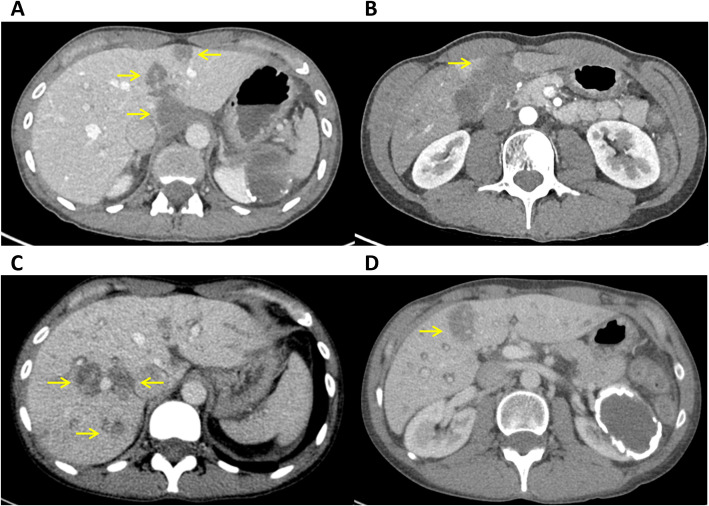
(A, B) Axial contrast-enhanced CT scans acquired within 24 hours after the first and (C, D) after the second histotripsy treatment session showing expected response in the treated lesions (yellow arrows).
